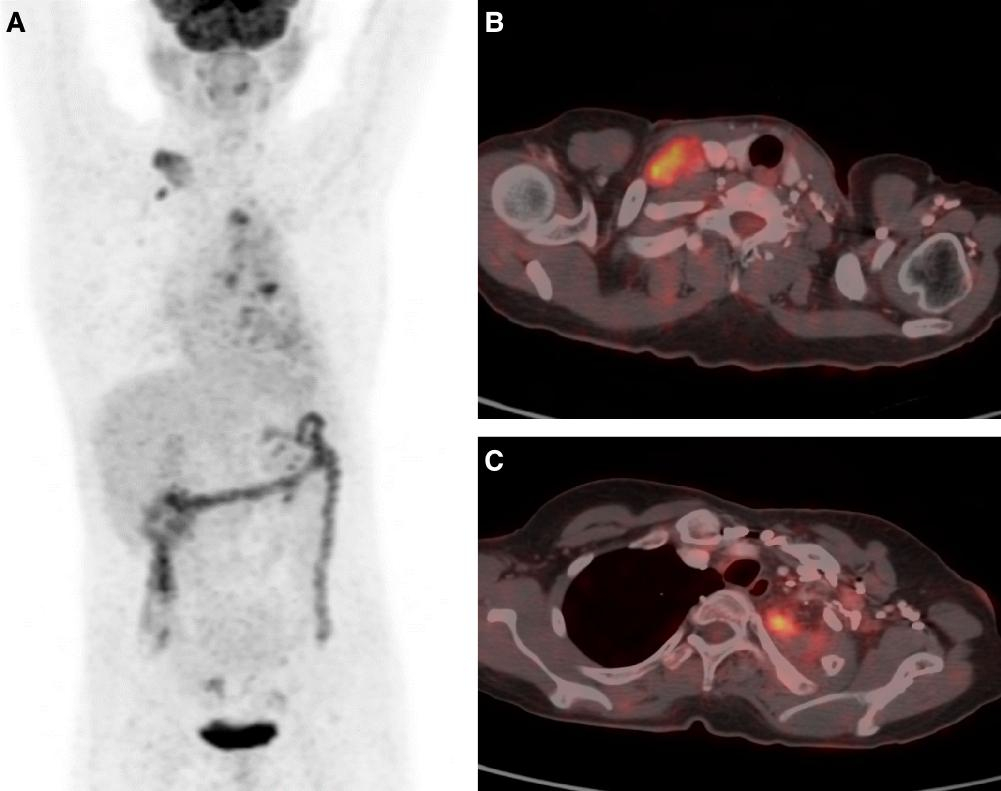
18 F Fluro deoxy glucose positron emission tomography computed tomography (18F FDG PET-CT) showing a whole-body planer image with normal tracer uptake in the brain and bladder, with some uptake in the cervical and thoracic regions (A). A few pleural-based, ill-defined lesions with a maximum standard uptake value (SUVmax) of 7.7 in the apex of the left lung and multiple nodular soft-tissue densities of varying sizes on the left lung (B). Tracer-avid enlarged right supraclavicular lymph nodes, the largest being 2.0 × 3.4 cm with an SUVmax of 8.2. Mildly tracer-avid left supra- and infraclavicular lymph nodes. Tracer-avid subcentimetric to centimetric lymph nodes at paratracheal left posterior intercostal locations (C).
